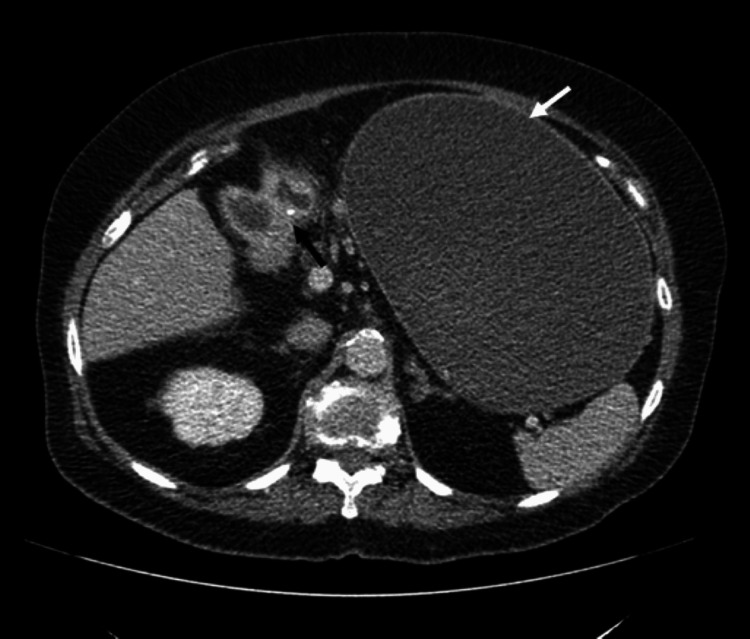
Axial portal venous CT shows gallbladder adherent to duodenum with a calcified opacity representing a gallstone within the duodenal lumen (black arrow) and massive gastric distension (white arrow).
